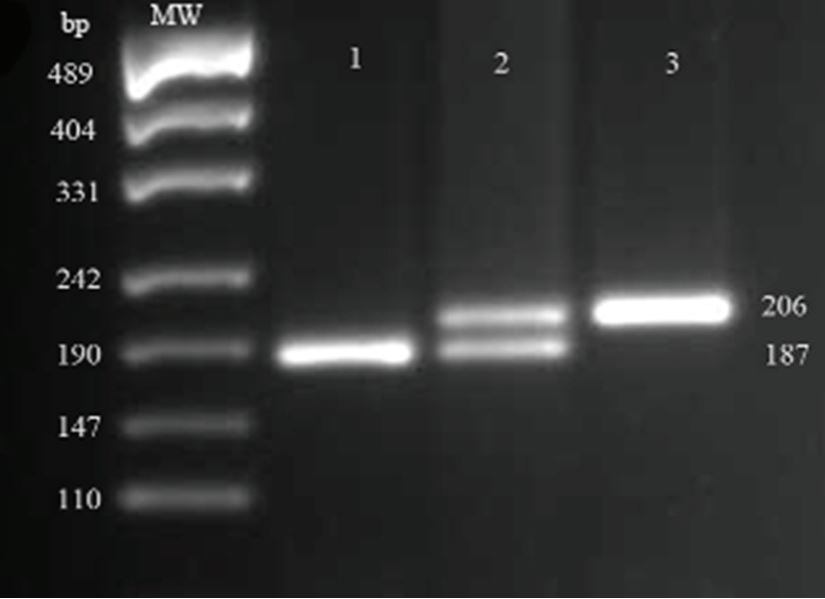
Results of Restriction Fragment Length Polymorphism (RFLP) using *RsaI *for detection of 1014C/T genetic variation in CCR4 gene on agarose gel 2.5%. TT homozygote, CT heterozygote, and CC homozygote samples have been run in lane 1, 2, and 3, respectively. MW: molecular size marker.
